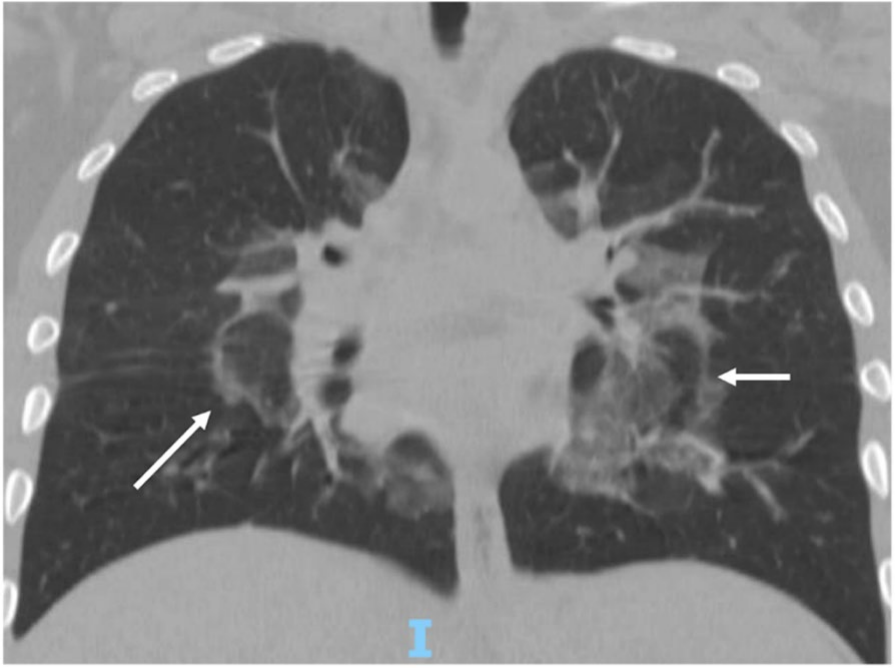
Chest CT (coronal view) showing the “atoll sign” or “reversed halo sign” bilaterally (arrrows)
